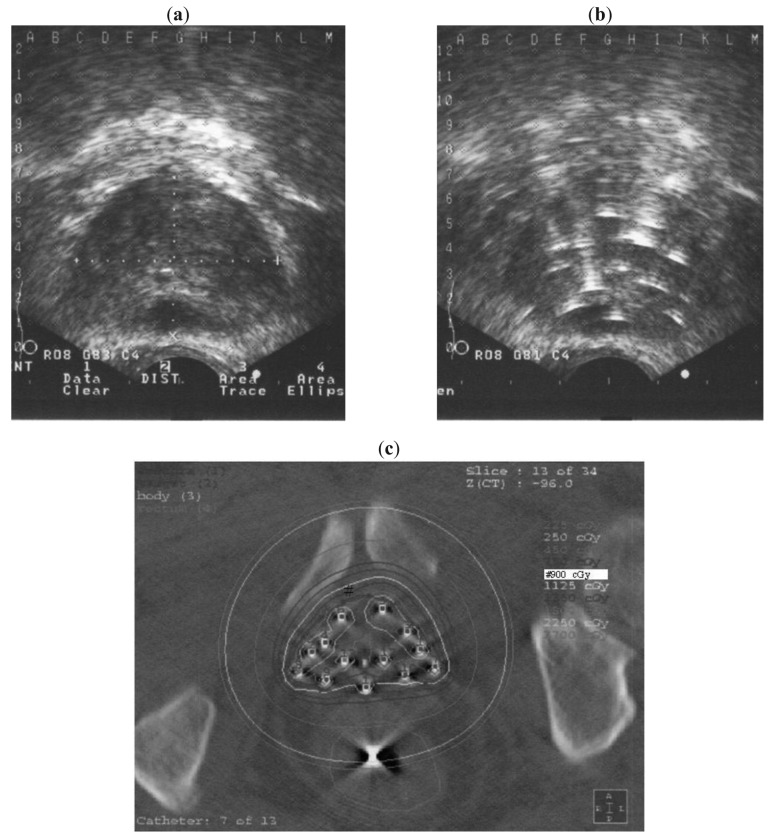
Axial trans-rectal ultrasound (TRUS) showing the axial largest cross-section of the prostate gland before (a) and after (b) insertion of applicator needles. (c) Axial view after computed tomography-based treatment planning reveals homogeneous radiation distribution to the whole prostate gland.
